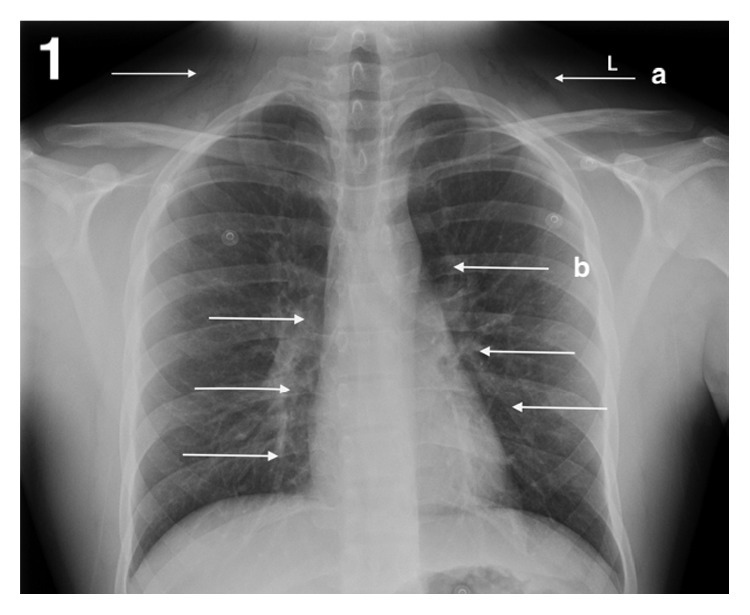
X-ray showing supraclavicular subcutaneous emphysema and mediastinal shadow, consistent with pneumomediastinum.
